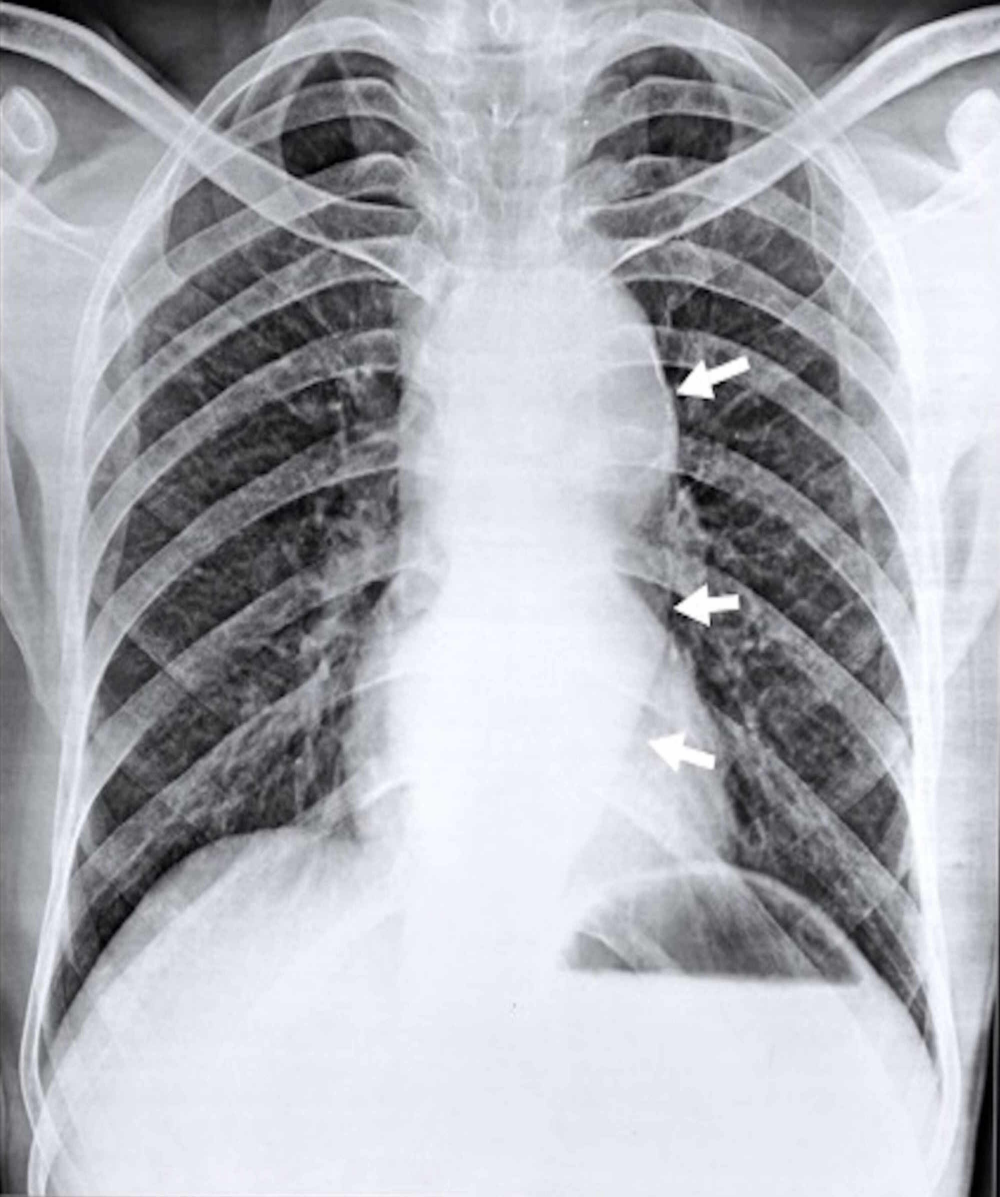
Chest X-ray (CXR) showing radiodense shadow at aortic knuckle and retrocardiac area (white arrows) with evidence of discontinuous linear calcification (upper white arrow).
